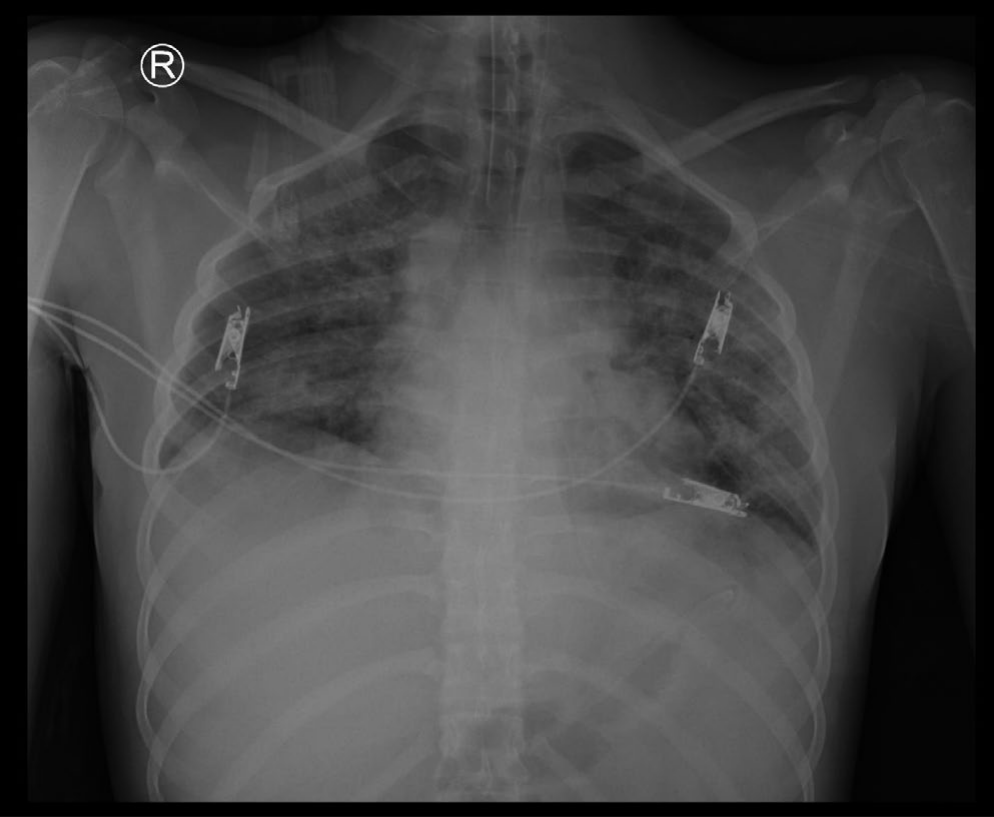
A frontal chest radiograph reveals bilateral patchy opacities throughout both lungs suggestive of ongoing infectious process
